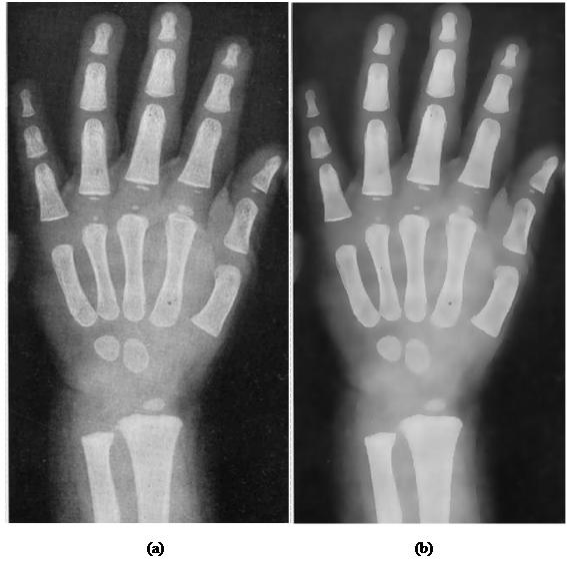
Comparison of radiographs before and after anisotropic diffusion. In this figure, a child's left hand bone radiograph is used to demonstrate the anisotropic diffusion effect: (a) Hand radiograph image before diffusion (b) Hand radiograph image after diffusion. As the result presents, the bone area has been smoothed to become homogenous area; the black holes and dots in have been filled by similar pixel intensity with the surrounding bone. Despite this filtering process, the edges of hand structure are preserved and can be clearly seen.
